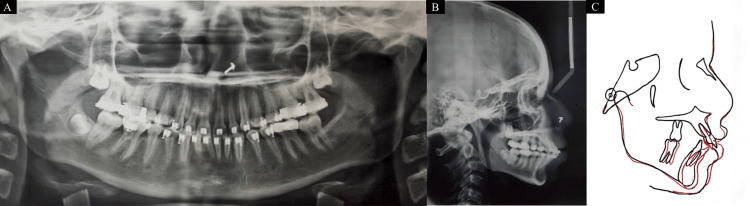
Current (A) orthopantomogram. (B) Lateral cephalogram. (C) Pre- and posttreatment superimpositions
